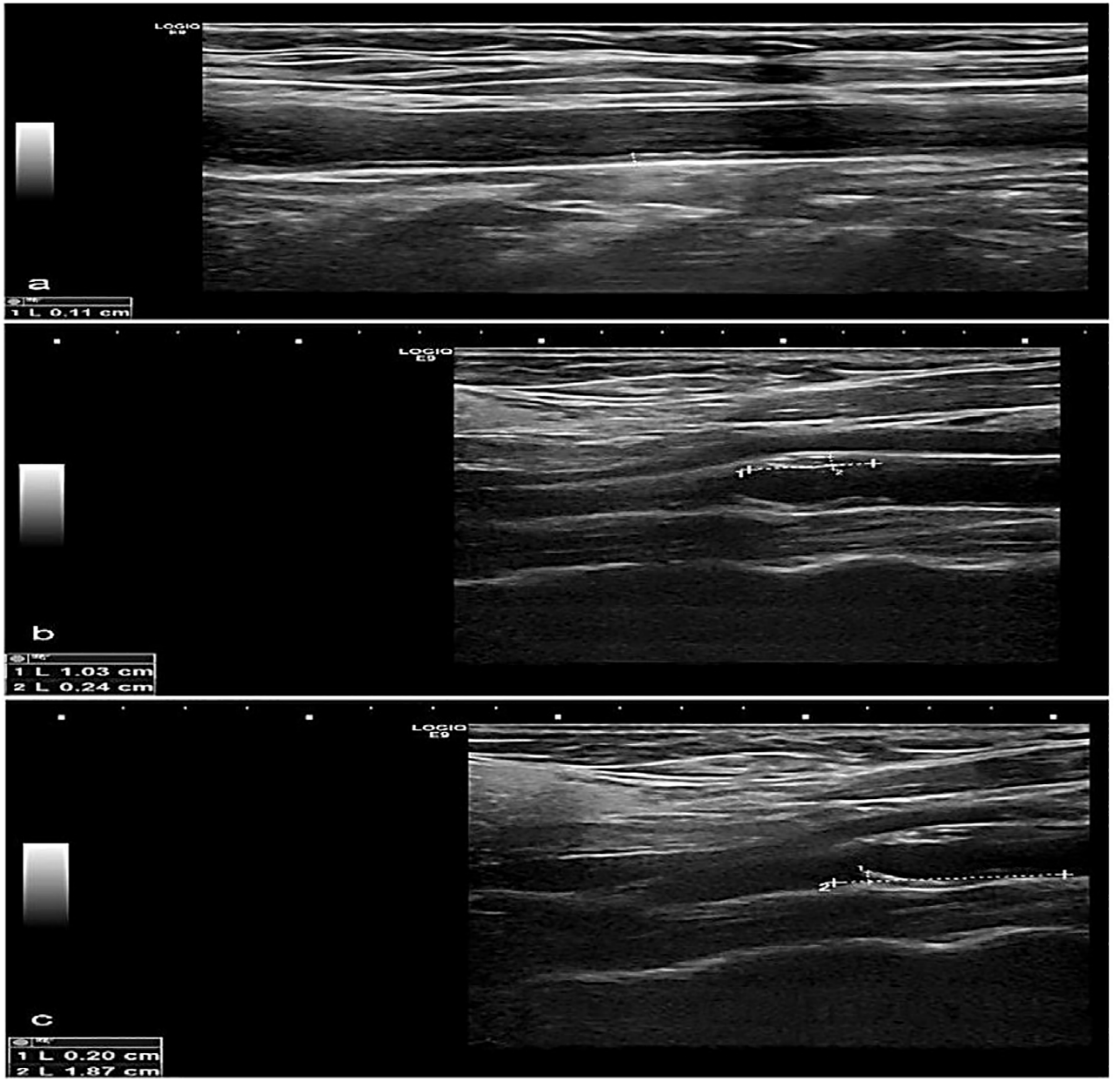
Greyscale longitudinal ultrasound images in a 70-year-old female diabetic and hypertensive patient showing (A) increased intimal/medial thickness at the right common carotid artery, (B) and (C) calcified plaques at opposing walls of the left carotid bulb.
